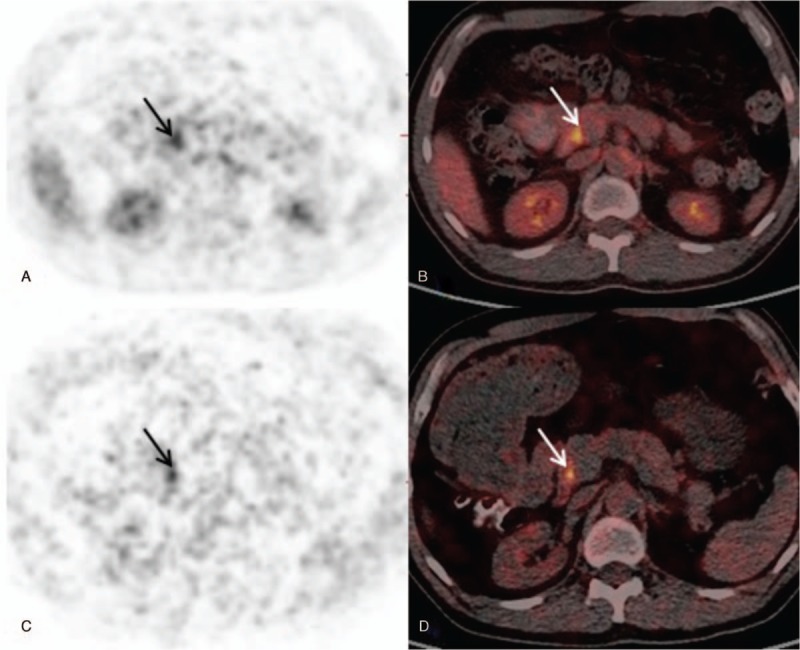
A benign duodenal lesion (adenoma) in a 45-year-old male shows ^18^F-FDG uptake on early (A, B) and delayed (C, D) PET/CT imaging. The SUV_max_ has a slight decrease from 5.2 on the early scan to 5.1 on the delayed scan. The range of delayed stage was smaller than earlier stage. ^18^F-FDG = fluorine-18 fluorodeoxyglucose, PET/CT = positron emission tomography/ computed tomography, SUV_max_ = maximum standardized uptake value.
